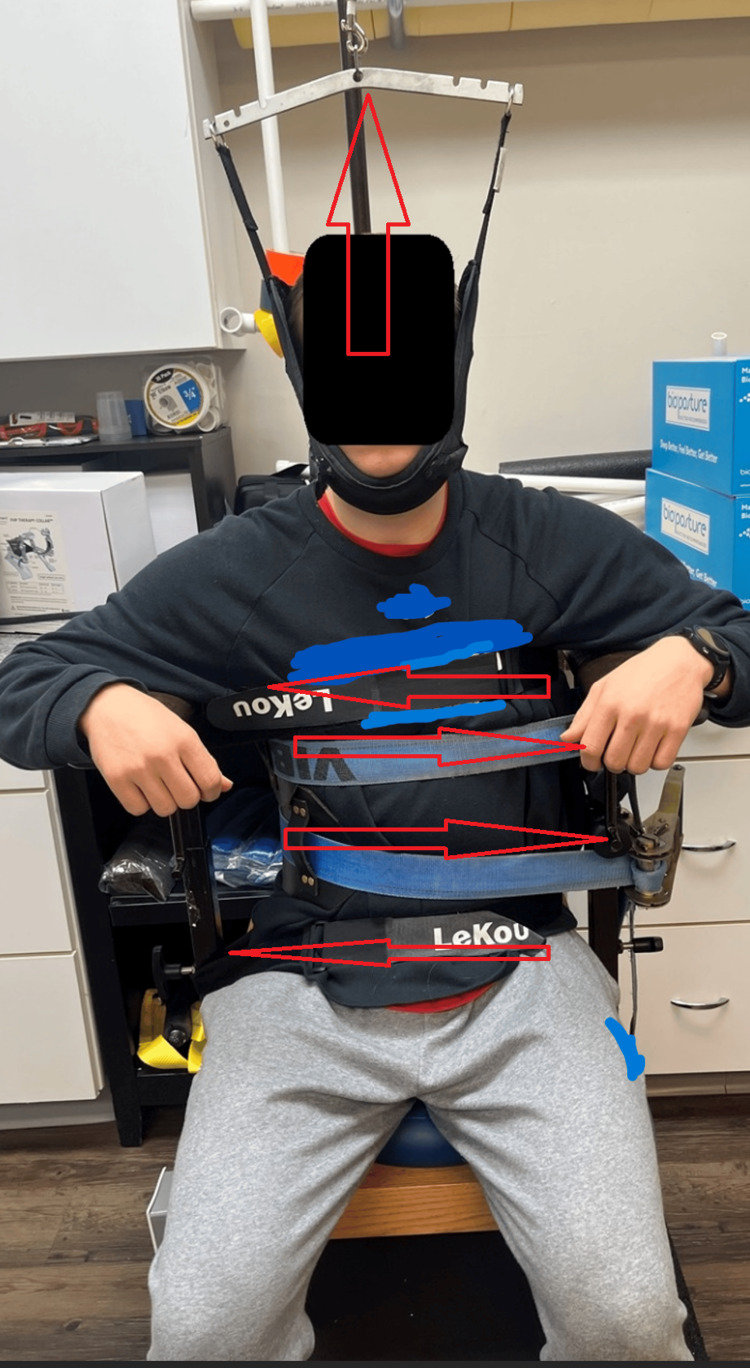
Scoliosis traction chair Scoliosis traction chair (mechanical traction) (whole-body vibration) (proprioceptive neuromuscular re-education) is a combination of whole-body vibration with mechanical traction with opposing forces for Cobb angle reduction. The patient is seated in the scoliosis chair with 10 pounds of Y-axis traction, lateral pulling straps to reduce the Cobb angles, and whole-body vibration with simultaneous vertical traction. Placement in the scoliosis chair is verified by X-rays. The purpose is meant for movement, balance, coordination, kinesthetic sense, posture for sitting, or standing activities. This was performed for 30 minutes each session (model used for demonstration).
